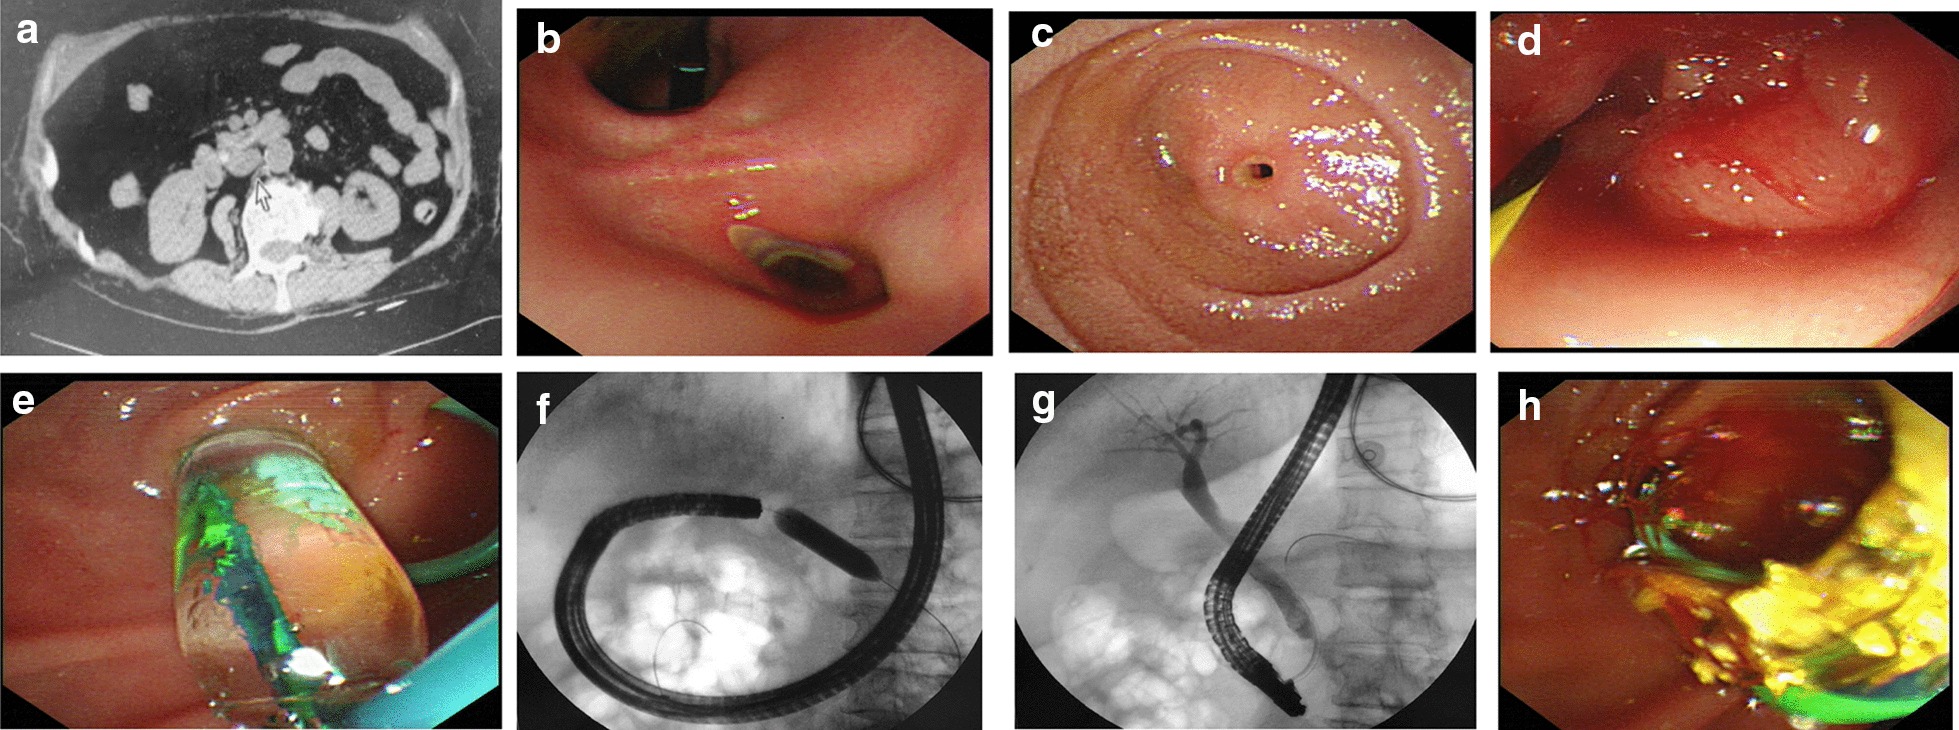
Imaging data for the patient. a Abdominal computed tomography showed a high density stone in CBD; b endoscopic view of gastric ulcer in pylorus; endoscopic appearance of a duodenal diaphragm with a 2 mm aperture, close view (c) and endoscopic dilatation view (d); e endoscopic view of balloon catheter in situ; f radiographic image of balloon dilatation. g After dilatation of diaphragm in the lumen, endoscopic tube passed through the stricture, ERCP radiography presented a 1 mm stone in CBD; h stone was pulled out through basket
